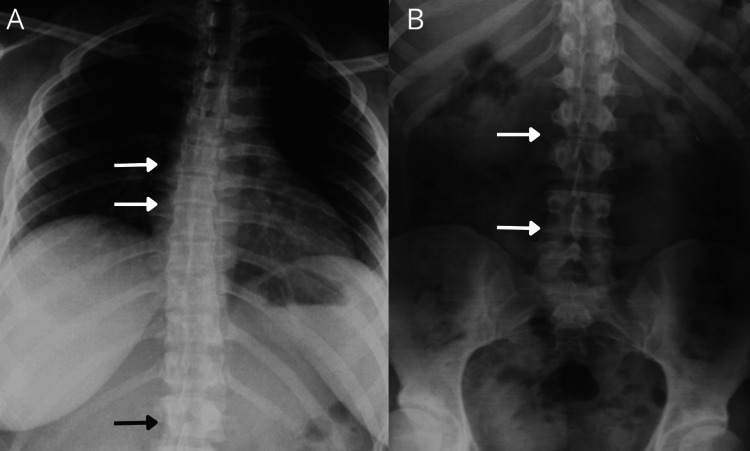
X-ray images of the spine with highlighted areas of curvature X-ray images showing mild scoliosis and pelvic asymmetry. (A) Dorsal spine in anteroposterior (AP) view, showing mild right convex scoliosis. (B) Lumbar spine in AP view, showing mild left convex scoliosis. White arrows indicate the regions of abnormal curvature
